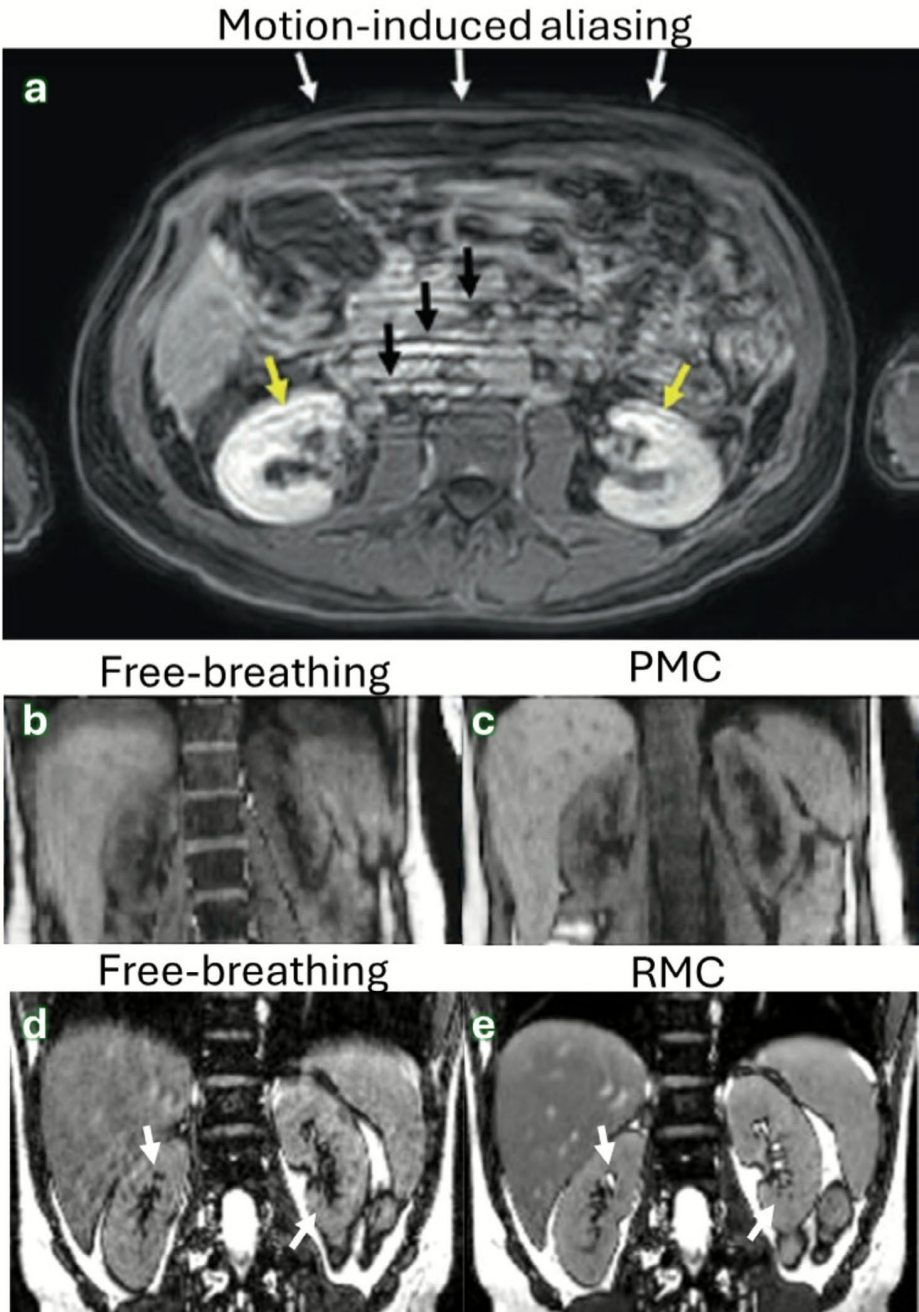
Breathing motion in liver and kidney MRI. (a) A failed breath‐hold in a Cartesian T1‐weighted Dixon GRE results in replicating the fat boundaries throughout the FOV (ghosting). (b) Free‐breathing 3D stack‐of‐stars T1‐weighted GRE scan demonstrates blurring, concealing liver and kidney boundaries. (c) Data from (b) prospectively motion corrected (PMC) using free‐induction decay echoes. (d) Blurring from radial imaging as in (b), now from a T2‐weighted acquisition. (e) Data from (d) retrospectively motion corrected (RMC). This approach incorporates all of the data into the reconstruction of a single image, achieving higher SNR. Adapted from [68].
